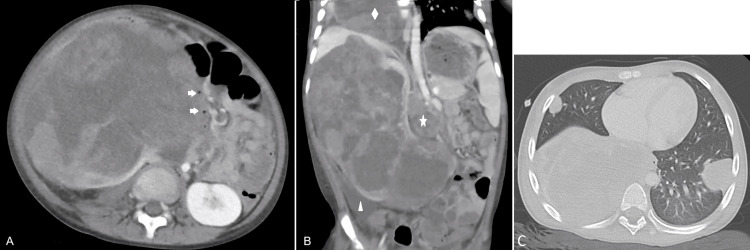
Preoperative CT scan CT: Computed tomography Figure 1. Right renal solid tumor with heterogeneous enhancement, including necrotic and hemorrhagic areas, and lung metastases A. Axial plane: The tumor compresses the inferior vena cava medially and displaces retroperitoneal structures. The duodenum is shifted to the left, nearly flattened, and contains blood with an air-fluid level (indicated by arrows). B. Coronal plane: Presence of precaval lymphadenopathy (indicated by a star). Free pelvic fluid with a density of 40 HU (triangle). The largest pulmonary metastasis measuring up to 9 cm in the right lower lobe (diamond). C. Thorax axial plane: Bilateral pulmonary metastases.
